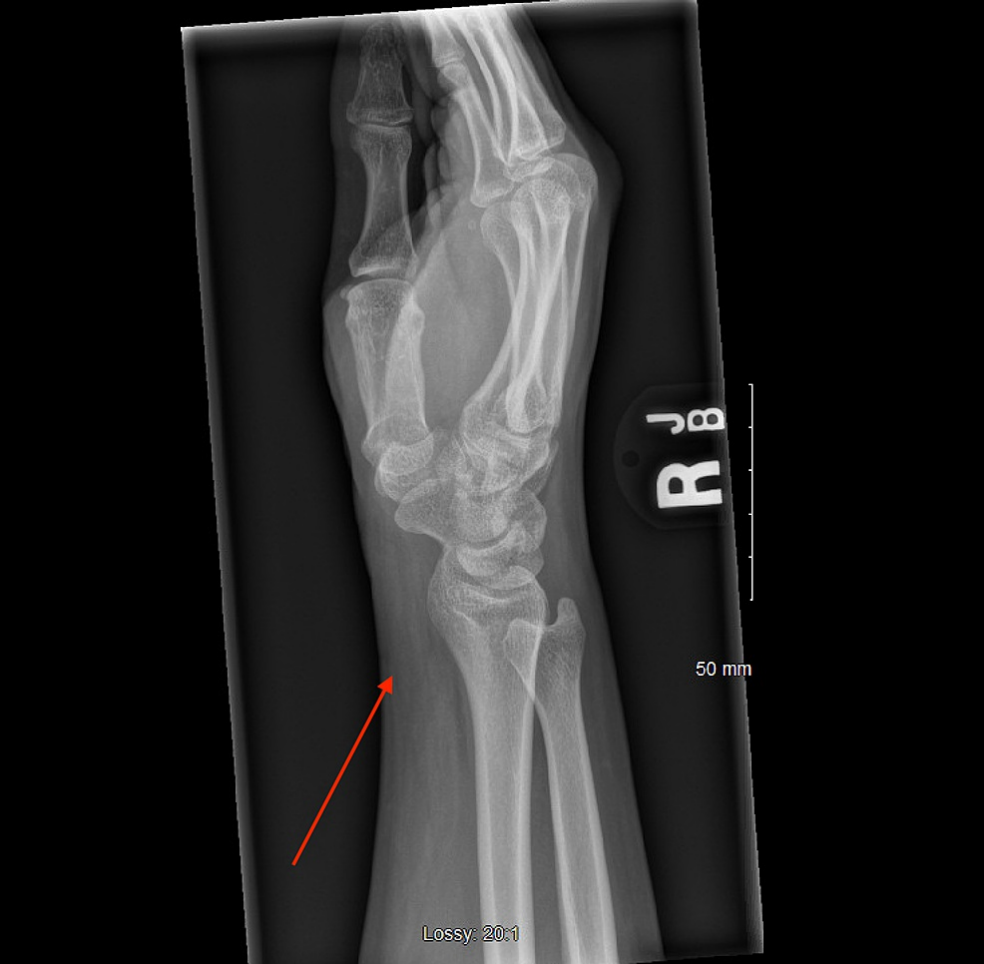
Lateral X-ray of patient's right wrist demonstrating moderate volar soft tissue swelling
